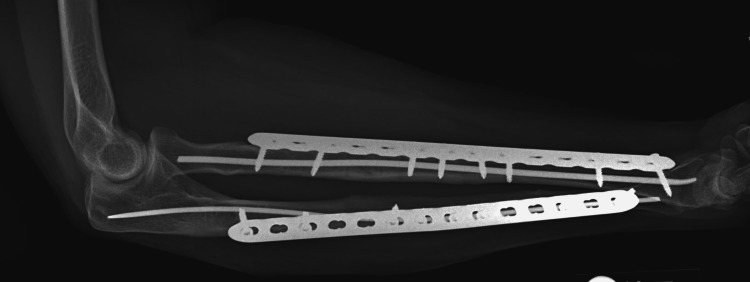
Lateral forearm radiograph at 18 months after definitive surgery.
